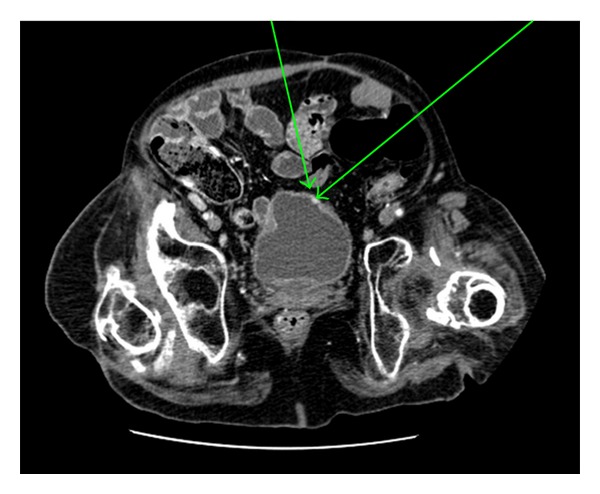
An arterial phase view of the carcinoid tumor seen on CT urogram. The tumor is located at the anterior portion of the cystoplasty along the bladder-ileum border (arrows pointing to area of concern for tumor).
